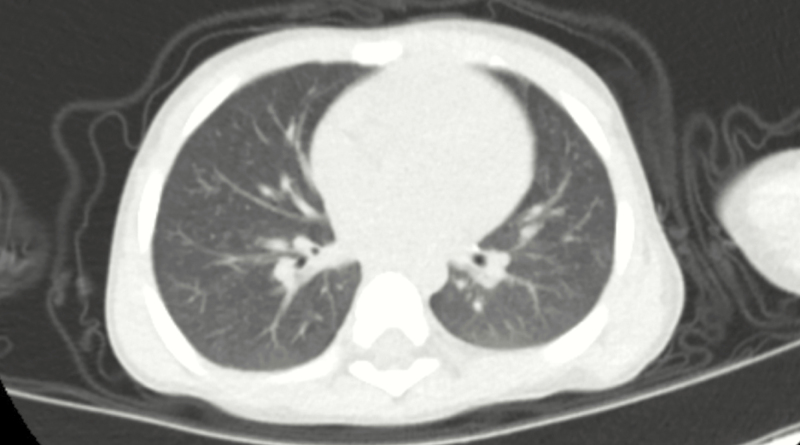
The chest CT manifestations of patient 3 showing plaques.
